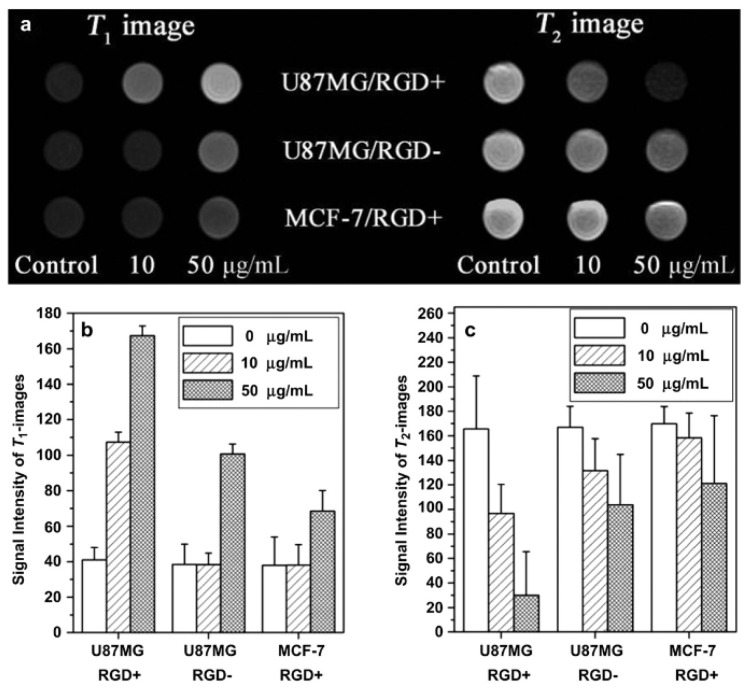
(a) T_1_- and T_2_-weighted MR images of Fe_3_O_4_@SiO_2_ (Gd-DTPA)-RGD NPs in U87MG and MCF-7 cells at different concentrations of NPs after incubation for 6 h on a 3T MRI system (RGD+: Fe_3_O_4_@SiO_2_ (Gd-DTPA)-RGD). RGD-: Fe_3_O_4_@SiO_2_(Gd-DTPA); (b) signal intensity analysis for T_1_-weighted MR images; (c) signal intensity analysis for T_2_-weighted MR images. Reproduced with permission from [117]. Copyright from Elsevier.
